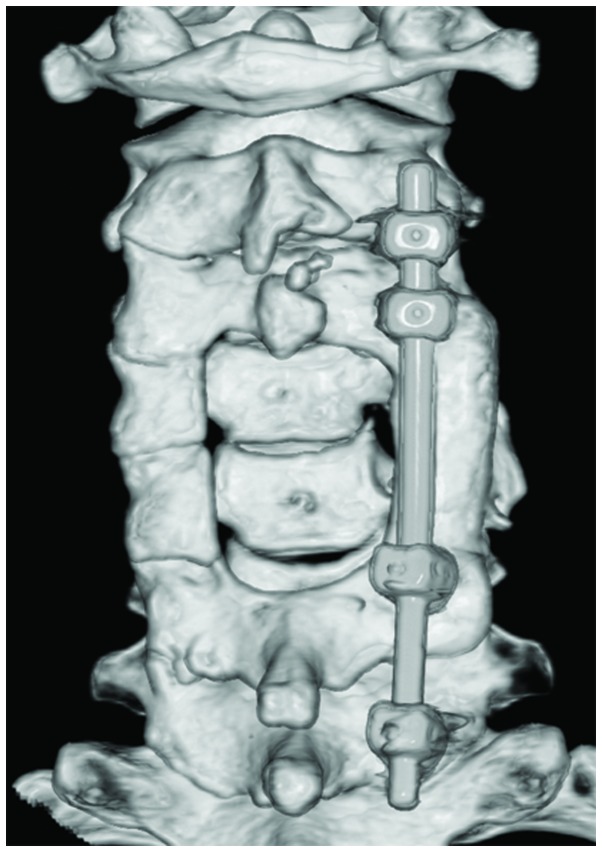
A 3-dimensional reconstruction of the CT scan showing successful fusion at 2 years post-surgery. CT, computed tomography.
